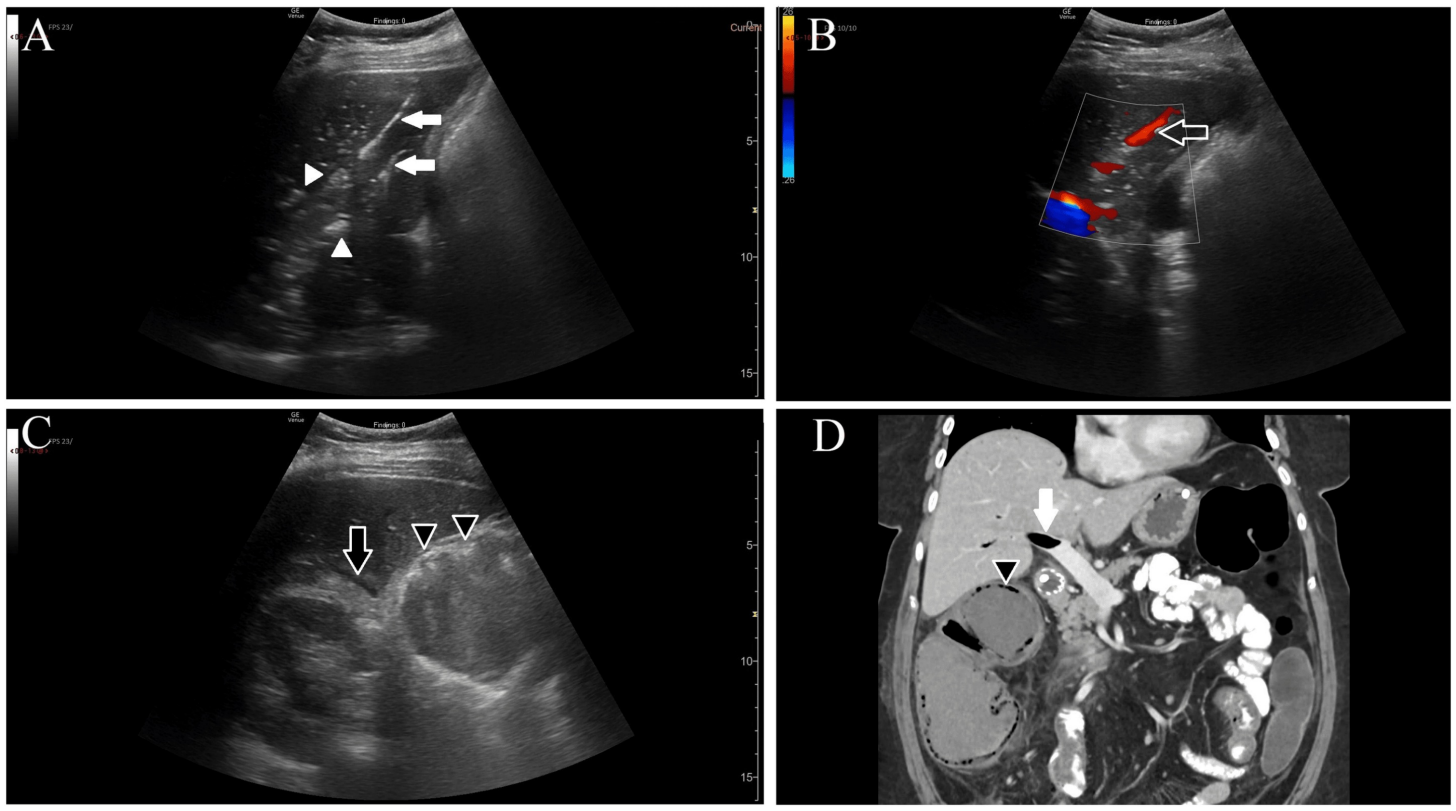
Case 1 - POCUS images and CT A: Hyperechoic collections of PVG (white arrowheads) are visualized in the liver parenchyma. Linear collections of gas (white arrows) are seen within portal veins coursing in the long-axis plane. B: Color Doppler imaging of the linear collection (black arrow) demonstrates flow within the structure, identifying it as a portal vein containing microbubbles. C: B-mode image demonstrates perihepatic free fluid (black arrow) and colonic pneumatosis (black arrowheads). D: CT image demonstrates gas (white arrow) within the portal vein and colonic pneumatosis (black arrowhead). B-mode: brightness mode; PVG: portal venous gas; POCUS: point-of-care ultrasound; CT: computed tomography
